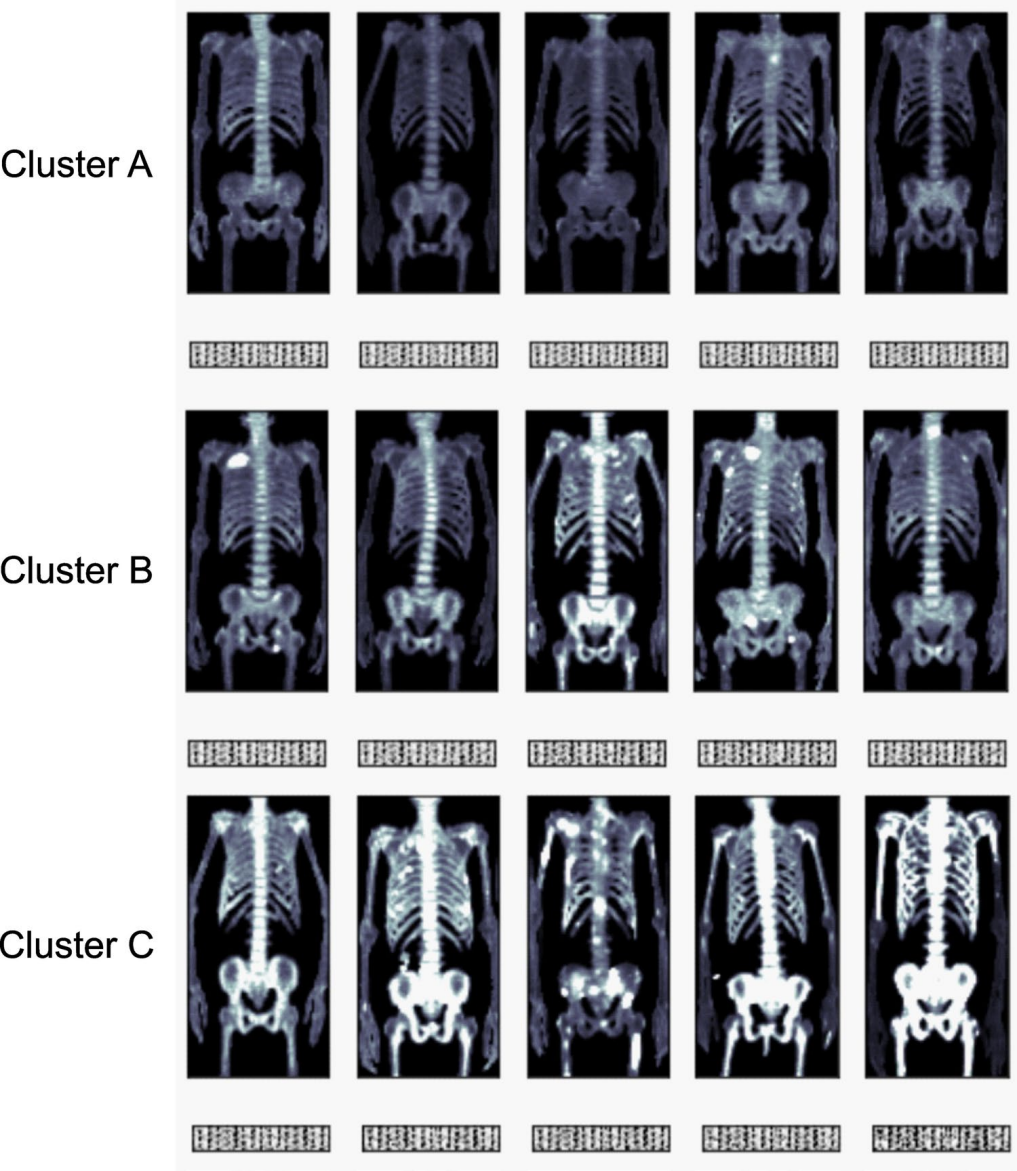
Representative anterior view MIP images of cases belonging to each cluster. Five representative cases are displayed for each cluster. The upper panels of each row are the MIP images of FDG PET/CT scans. The lower panels of each row are the encoded images showing the extracted features. There are visually apparent increases in FDG uptake intensity from clusters A to B and from B to C. *MIP* maximum intensity projection.
